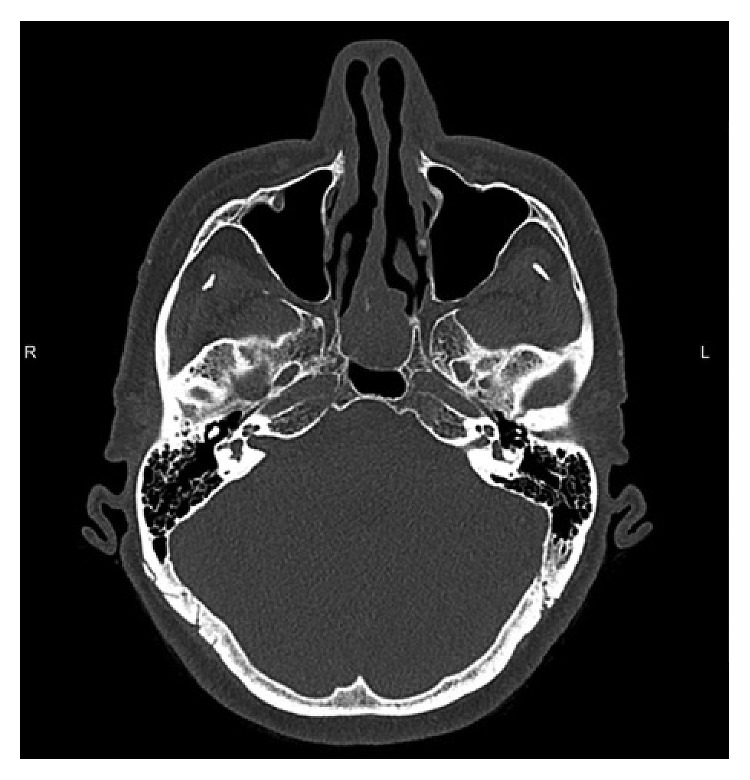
Axial CT image showing a soft tissue mass centered at the posterior nasal septum with extension into the nasopharynx.
